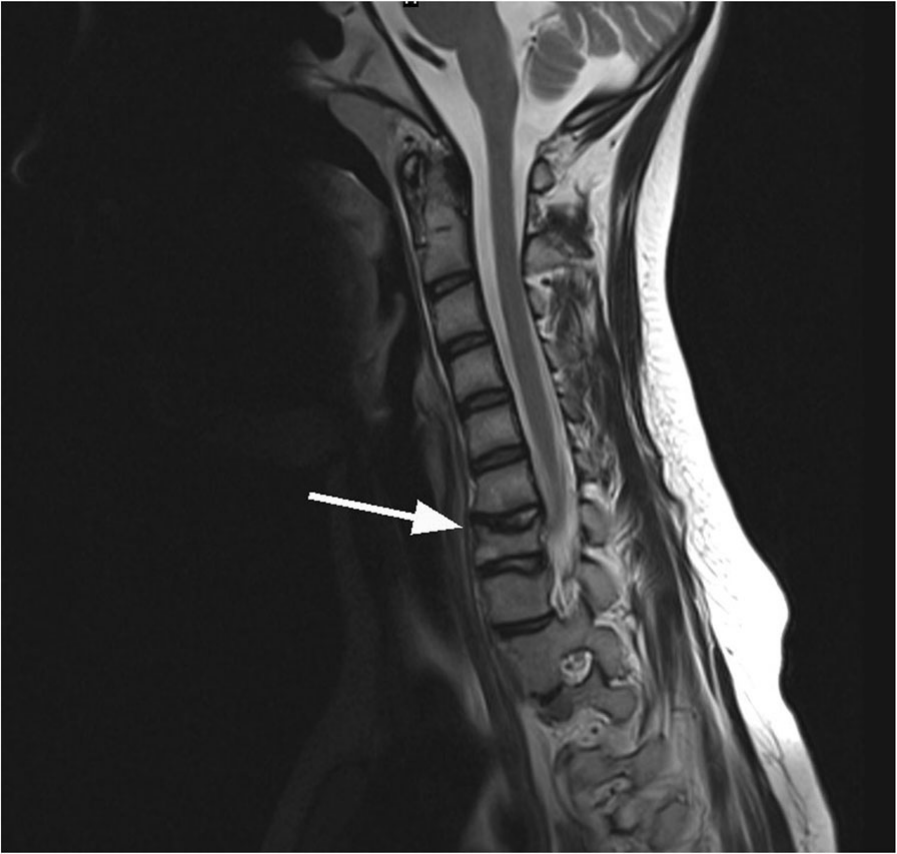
MRI of the patient’s neck. Destruction and lipoid degeneration in the seventh neck vertebrae is indicated by a white arrow
